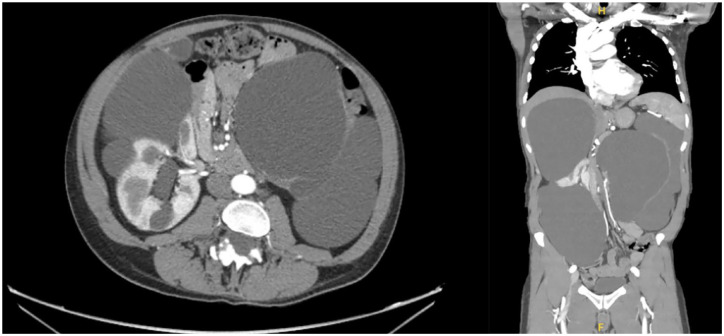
Computed tomography imaging reveals enormous bilateral kidney cysts. There are multiple bilateral extremely large renal cysts, some of which demonstrate thin internal septations without calcifications or nodularity. The cysts are predominantly homogeneous with predominantly fluid attenuation. The largest on the right measures 19 cm in craniocaudal extent. The largest peripheral cyst on the left measures 12 cm. There is resultant mass effect and displacement of the adjacent spleen and liver parenchyma as well as the small bowel mesentery. The spleen is enlarged at 15 cm. The central fluid-filled structure in the left kidney may be a markedly distended collecting system. The left renal parenchyma is markedly thinned and barely perceivable. The right renal pelvis is mildly dilated as well as a moderately distended pole calyx. Both ureters and urinary bladder are within normal limits. The partially visualized aspect of the pancreatic parenchyma is within normal limits.
